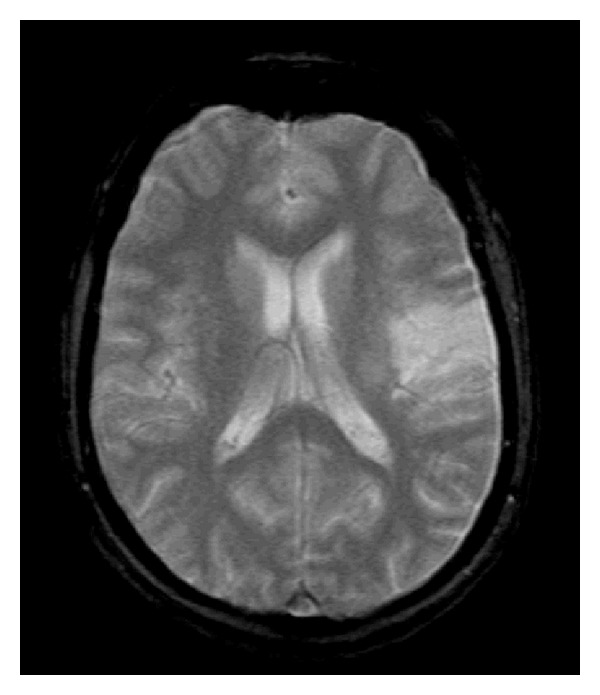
T2WI-MRI scan after one year demonstrating regression of the previous infarction.
